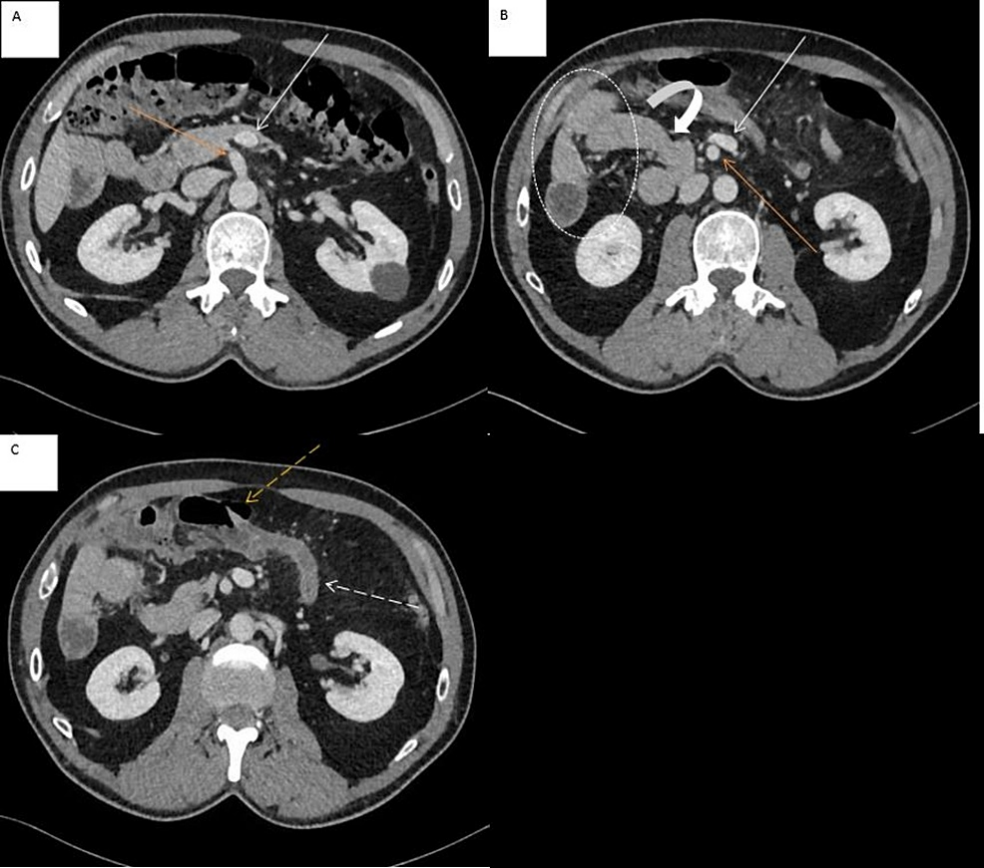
Multiple sections of axial contrast-enhanced CT abdomen. Axial contrast-enhanced CT abdomen showing SMA (orange arrow in A & B) to the right of SMV (white arrow in A & B), duodenojejunal junction to the right of the midline (curved arrow in B), small bowel jejunal loops on the right (dashed circle in B), and the cecum in the midline supraumbilical position (dashed orange arrow in C) with the arising inflamed appendix (dashed white arrow in C). CT: computed tomography; SMA: superior mesenteric artery; SMV: superior mesenteric vein
